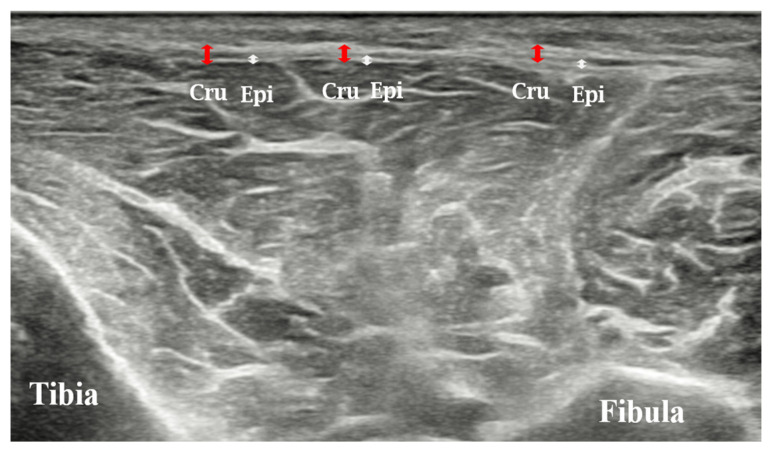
Ultrasound image of the thickness values of crural fascia and epimysial fascia of the tibialis anterior muscle. Red arrows indicate the thickness of crural fascia, and white arrows indicate the thickness of the epimysial fascia. Abbreviations: Cru, crural fascia; Epi, epimysial fascia.
